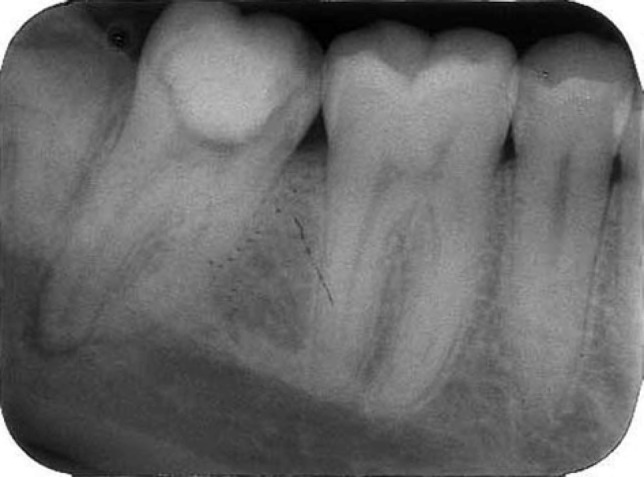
A pulpotomized right mandibular second molar. Careful examination of the radiographs revealed the possibility of more than two roots
